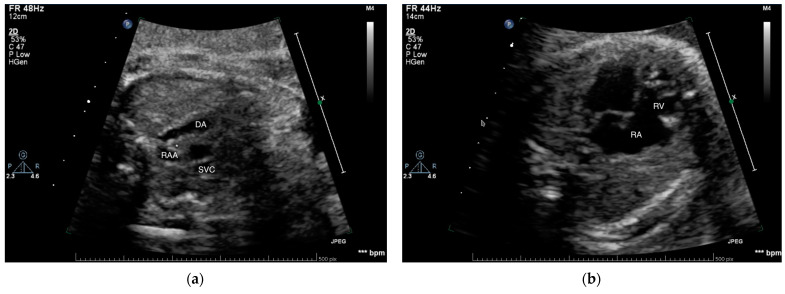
Images of fetal echocardiography at 20 weeks of gestation: (a) Transverse gray-scale sonogram of the fetal thorax showing aortic arch (RAA) coursing to the right of trachea (*), ductus arteriosus (DA) and superior vena cava (SVC), (b) transverse gray-scale sonogram of the fetal heart showing enlarged right atrium (RA), small right ventricle (RV), and apically placed dysplastic tricuspid valve.
